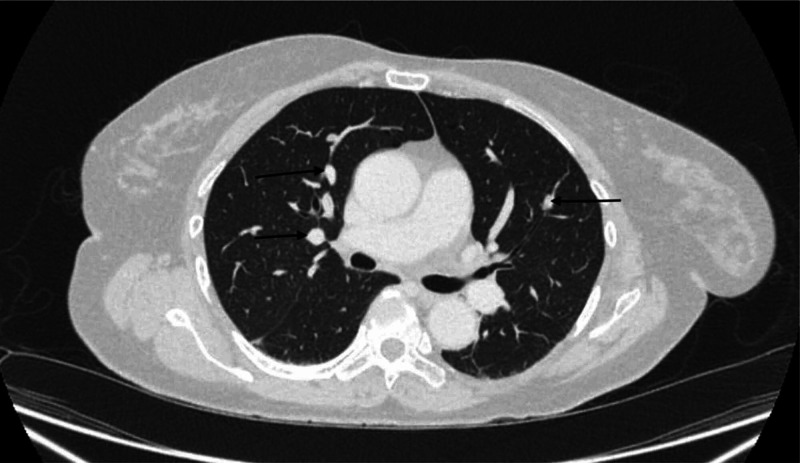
Computed tomography of chest showing multiple pulmonary nodules (black arrows) prior to starting everolimus 10mg PO.
